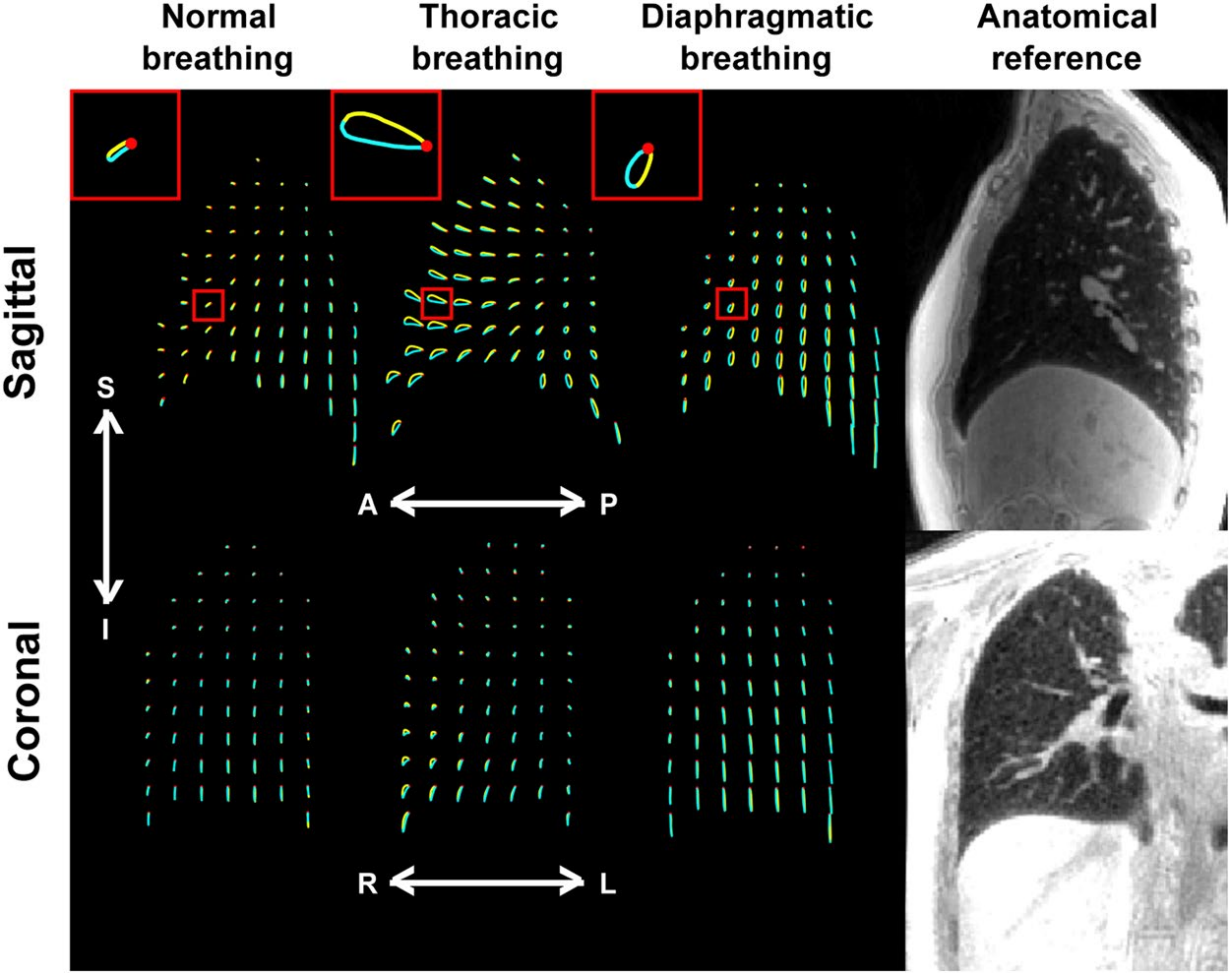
Sagittal (top) and coronal (bottom) views of the trajectories followed by elementary volumes of the right lung parenchyma, located at red dots positions at the end of expiration. For the sake of clarity, only one percent of the trajectories is shown. These trajectories are pictured for the normal (left), thoracic (middle) and diaphragmatic (right) breathing patterns. In each trajectory, the inspiration phase is represented in yellow and the expiration phase is represented in blue. In the top left-hand corner of each represented breathing pattern, a focus on one representative trajectory (location depicted by the small red squares) is given. A UTE MR image is given on the right of each row as an anatomical reference.
